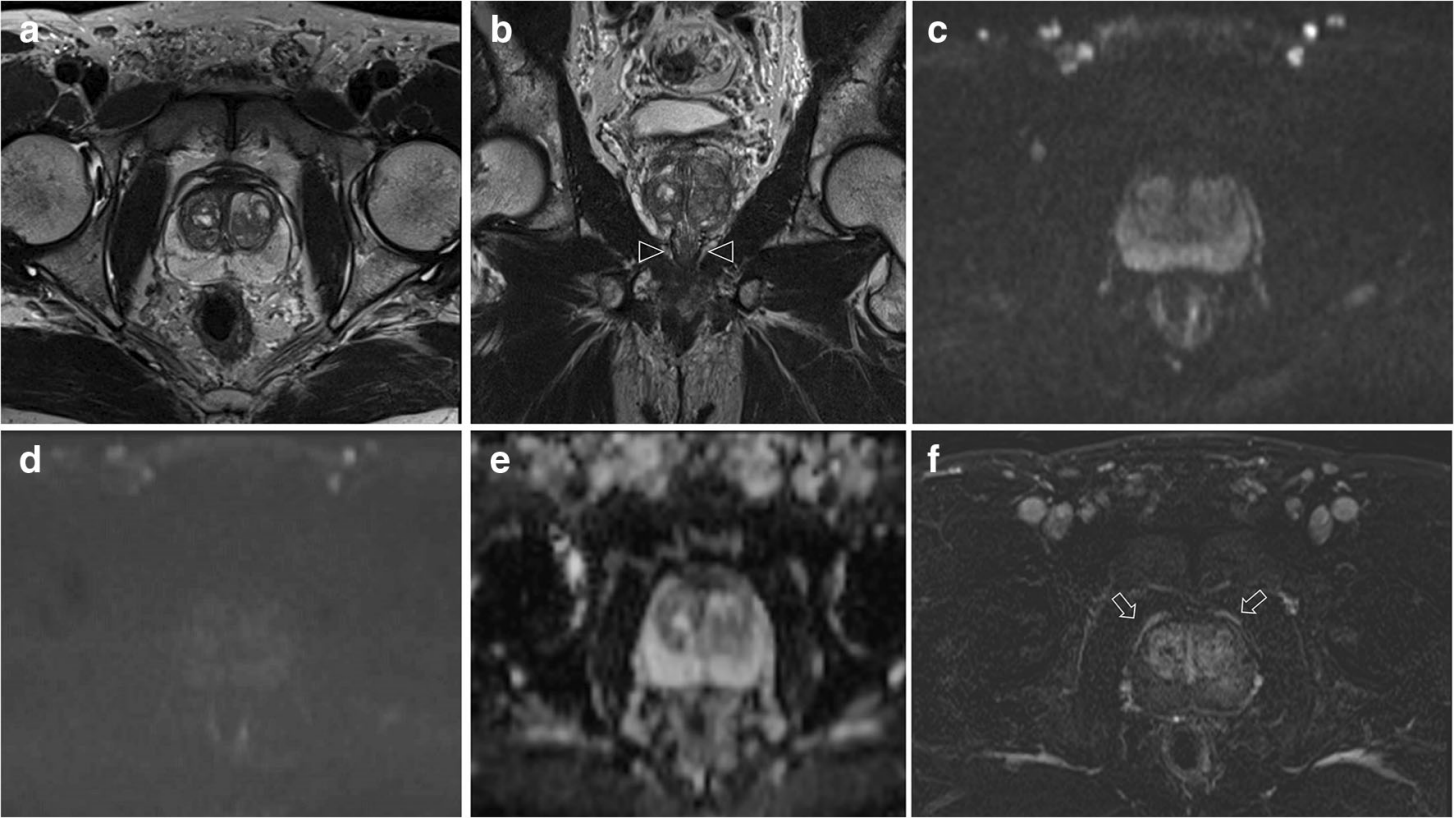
Images in a 50-year-old patient scanned on a 3-T MR system with a presenting PSA of 5 ng/ml and a prostate volume of 80 cc (PSA density: 0.06 ng/ml/ml), and bilateral Gleason 3 + 4 at biopsy. Axial (a) and coronal (b) T2-weighted images were judged of adequate diagnostic quality, and the arrowheads in (b) indicate the internal urethral sphincter clearly demarcated. The *b* = 1000 s/mm^2^ (c) and the high *b* sequences (*b* = 1400 s/mm^2^) (d) along with the apparent diffusion coefficient (e) map from diffusion-weighted imaging were of acceptable diagnostic quality but the in-plane resolution of (d) and (e) was deemed suboptimal by both readers. The subtracted dynamic contrast-enhanced sequences (f) were of adequate diagnostic quality (the arrows indicate the capsular vessels clearly demarcated) but the temporal resolution was 20 s (i.e., above the threshold of 10 s as per PI-RADS v.2.0 guidelines). The PI-QUAL score for both readers was 4 (i.e., two or more mpMRI sequences are independently of diagnostic quality). Final pathology confirmed bilateral organ-confined Gleason 3 + 4 (pT2c), with an overall tumour volume of 0.7 cc
